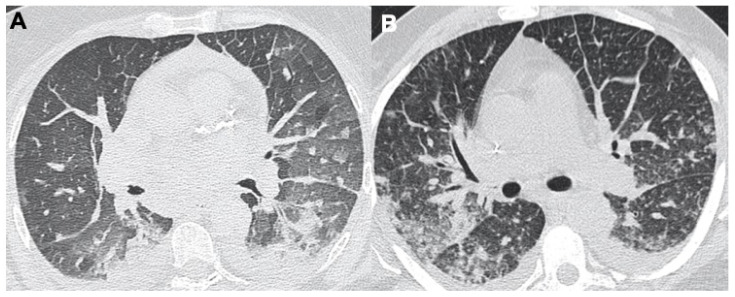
Two cases of congestive cardiac failure in COVID-19 patients. (A) Chest CT shows a bilateral interlobular septal thickening, cardiomegaly, pericardial effusion, bilateral pleural effusion, and broncho-vascular ground-glass opacities. (B) Bilateral pleural effusion, pulmonary interstitial oedema (interlobular septal thickening) and initial pulmonary alveolar oedema on the right thorax.
